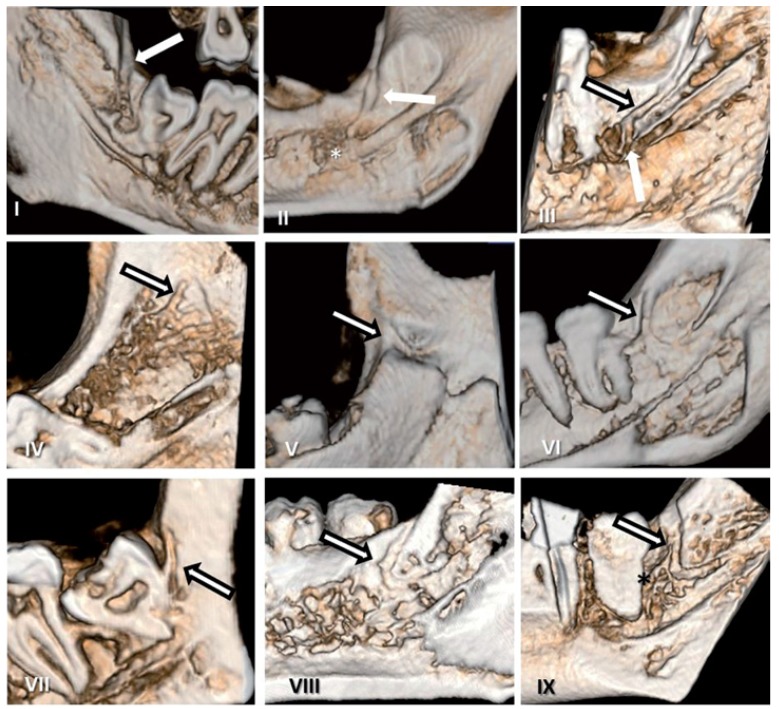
Corresponding sagittal CBCT cut of patients (which were shown in figure 2) indicates 3-D view of retromolar canal and its course.
